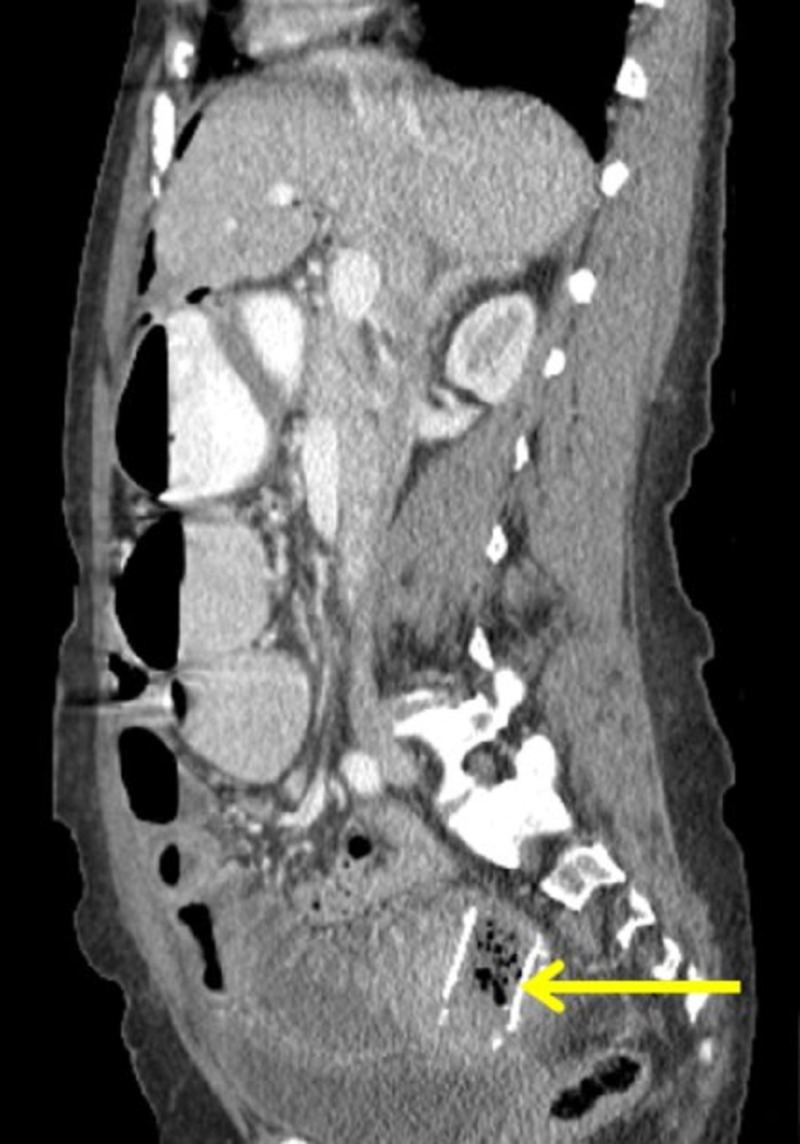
Computed tomography abdomen showing esophageal migrated stent-related perforation of the jejunal part of the small bowel; multiple loops of small bowel measuring up to 4.5 cm were noted (Coronal view). Arrow demarcates the location of the migrated stent.
